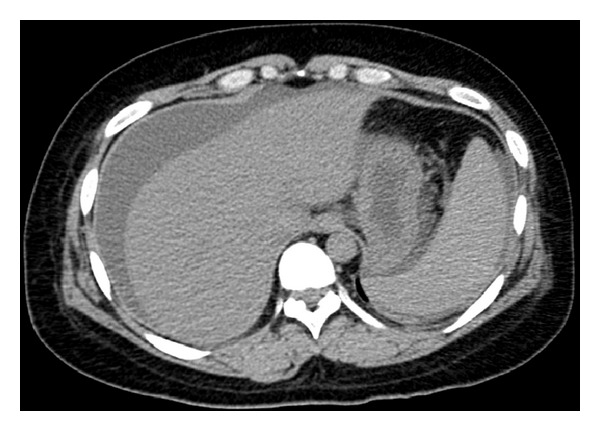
Computed tomography on admission showed atrophic liver and mild splenomegaly. There was moderate ascites but no evidence of cirrhosis.
